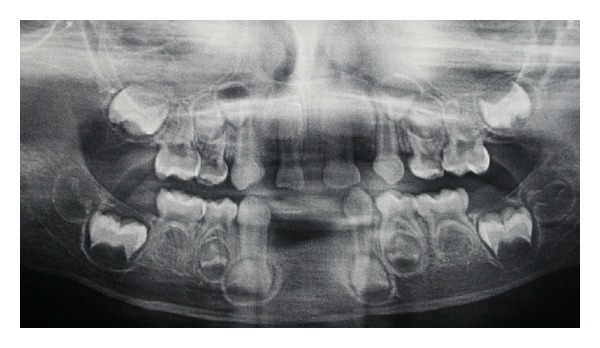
Panoramic radiograph demonstrating dental agenesis of 52, 62, 71, 72, 81, 82 (primary dentition) and 12, 22, 31, 32, 41, 42, 15, 25, 35, 45 (permanent dentition).
